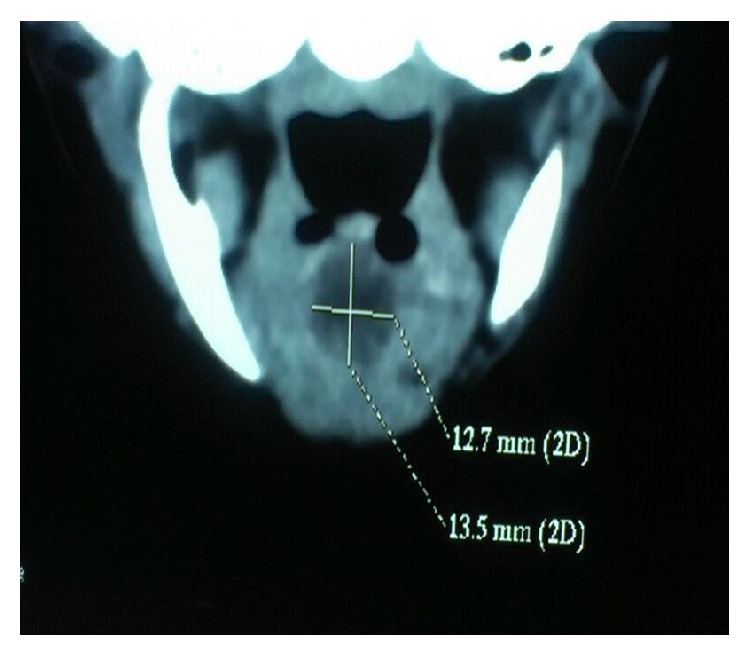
Contrast enhanced computed tomographic scan showing nonenhancing cystic lesion located at the base of the tongue measuring 12.7 mm × 13.5 mm.
